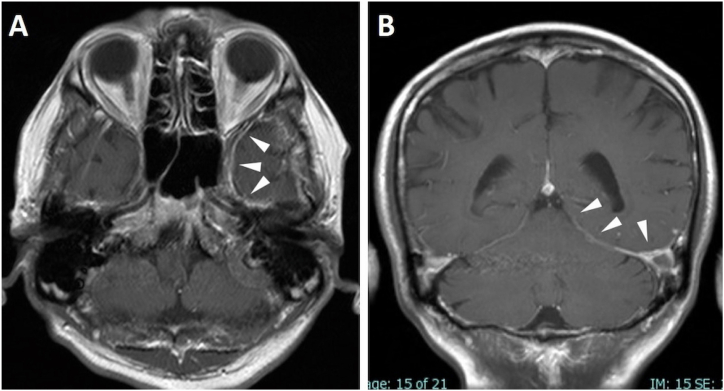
T1-weighted gadolinium-enhanced magnetic resonance imaging (MRI) of the brain at diagnosis. Enhancement and thickening of dura matter were observed predominantly on the left tentorium with horizontal (A) and coronal (B) view (arrows).
